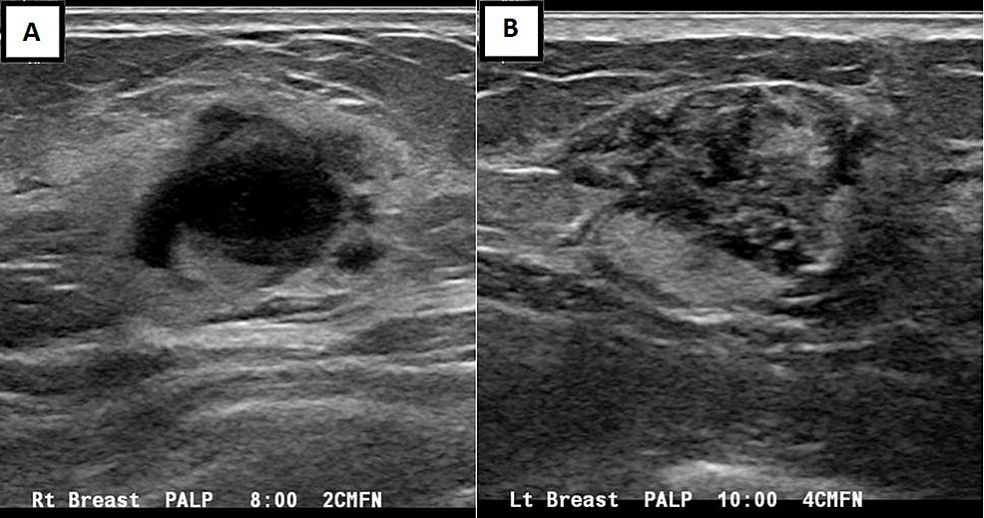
Bilateral breast ultrasonography Similar masses are seen in the right (A) and left (B) breasts with a complex hypoechoic central portion and a hyperechoic peripheral halo.
